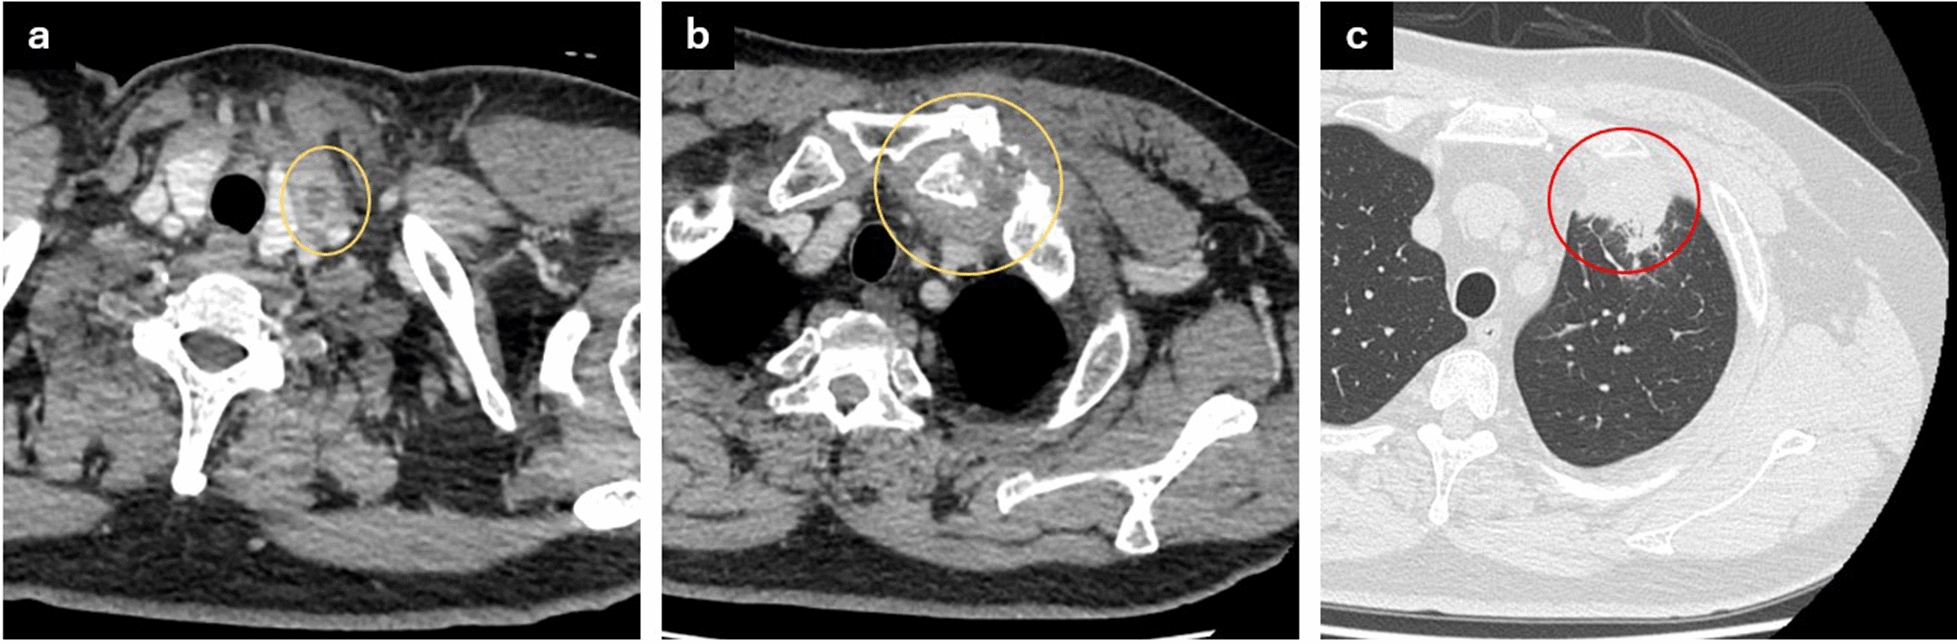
Contrast-enhanced computed tomography findings at the initial presentation. a Neck abscess (yellow circle), b soft-tissue swelling around the left sternoclavicular joint with clavicular bone destruction (yellow circle), and c infiltrative shadows in the left lung apex suggesting the spread of inflammation to the lung (red circle)
